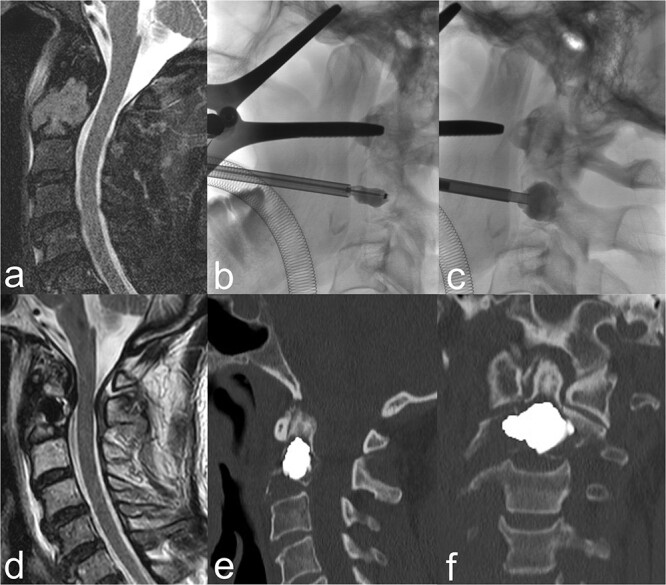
(a) The sagittal heavily T2-weighted fat-suppressed MRI imaging shows myeloma-related osseous destruction/pathological fracture of the corpus vertebrae axis. (b) BKP via a transoral approach. (c) After removal of the balloon catheter, the cavity created is filled with PMMA cement. (d) Centrally located cement plug in the sagittal T2-weighted MRI slice. (e) and (f) The sagittal and coronal reformed CT slices show a cement plug located centrally in the lesion. Cement leakage can be ruled out.
